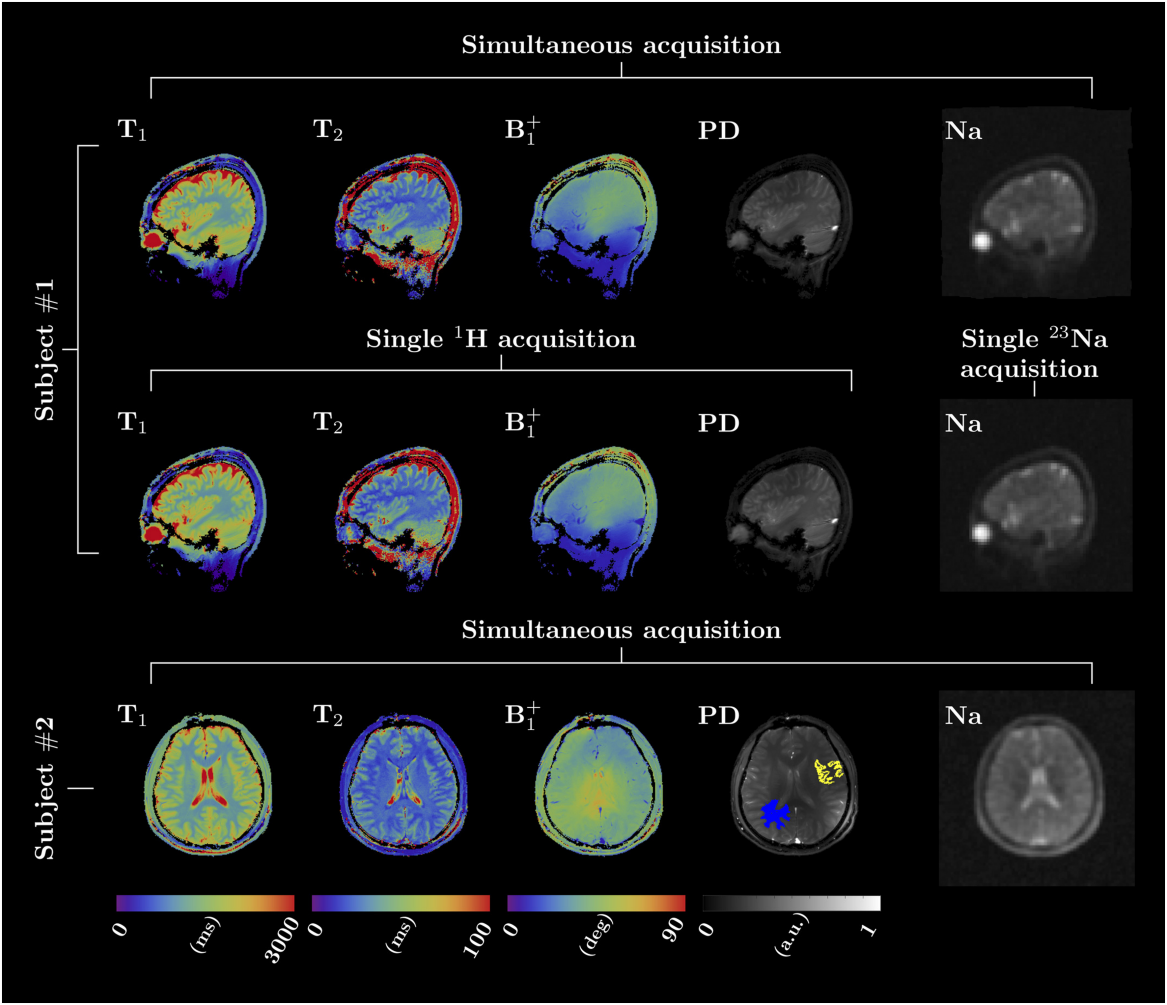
Proof of concept for simultaneously acquired ^23^Na MRI and ^1^H MR fingerprinting used to generate
